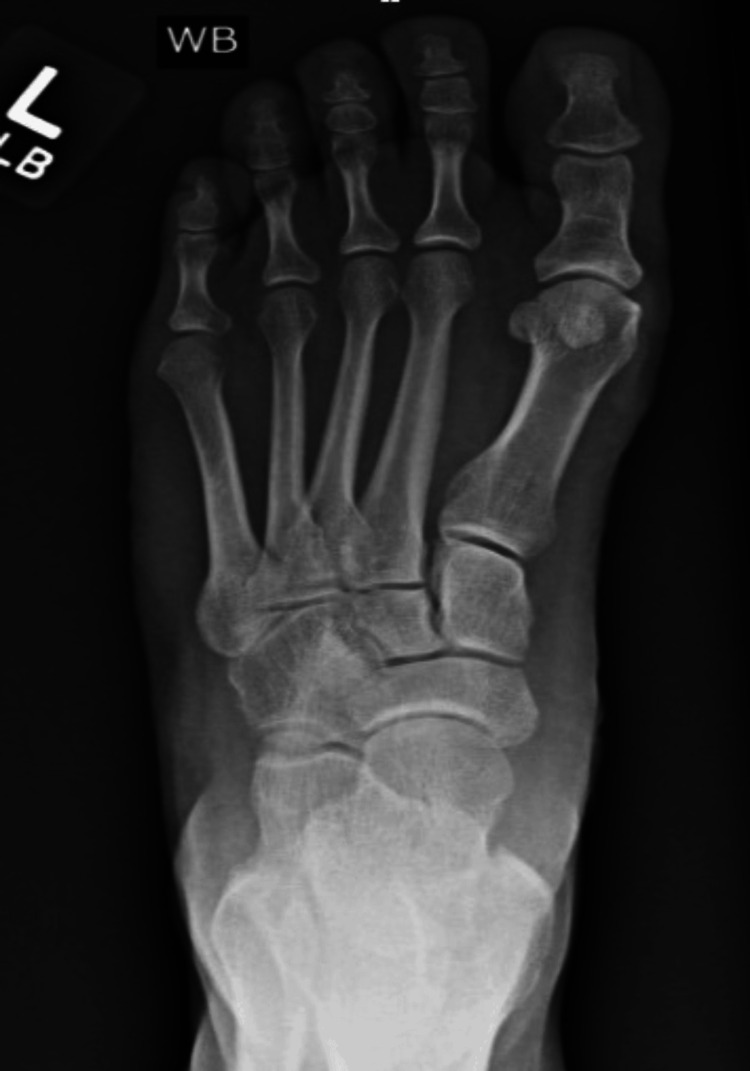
Preoperative weight-bearing radiograph demonstrating left hallux valgus deformity
